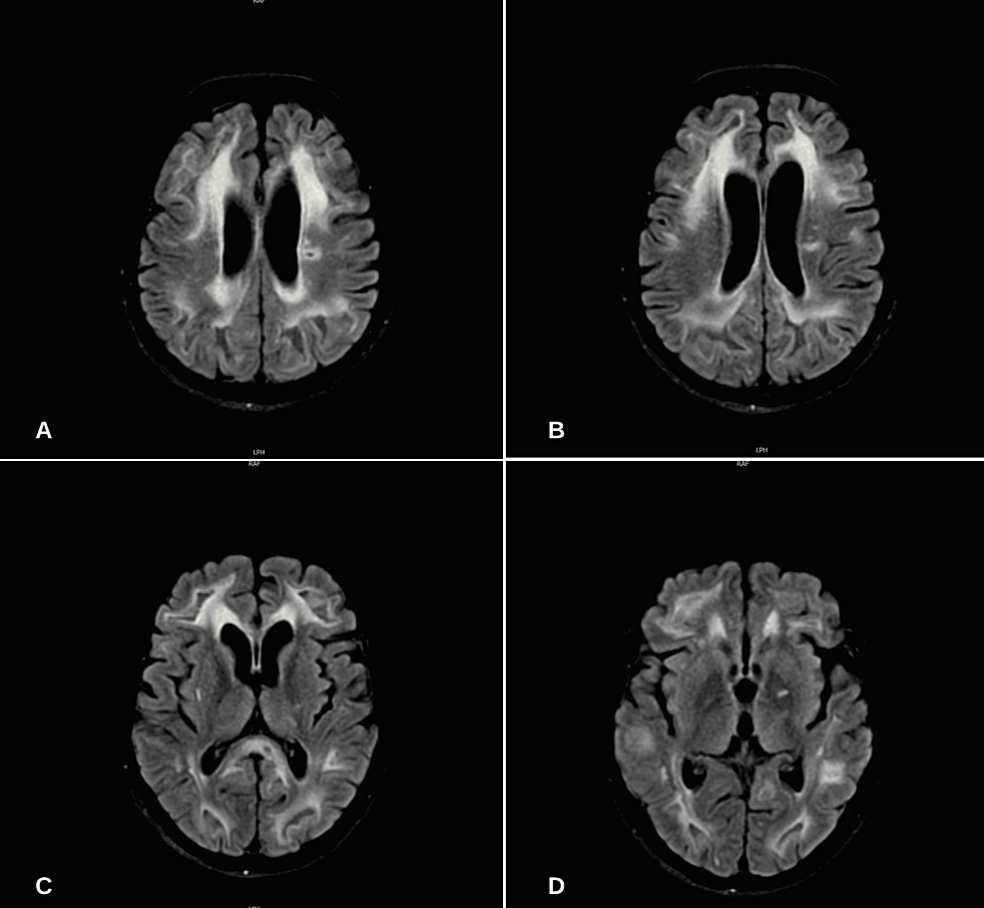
MRI brain T2-fluid attenuated inversion recovery (FLAIR) axial view obtained during week seven of the admission A-B: Chronic evolution of T2-hyperintensities in both bilateral frontoparietal and occipital cortices; C-D: Chronic evolution of T2-hyperintensities in subcortical structures
